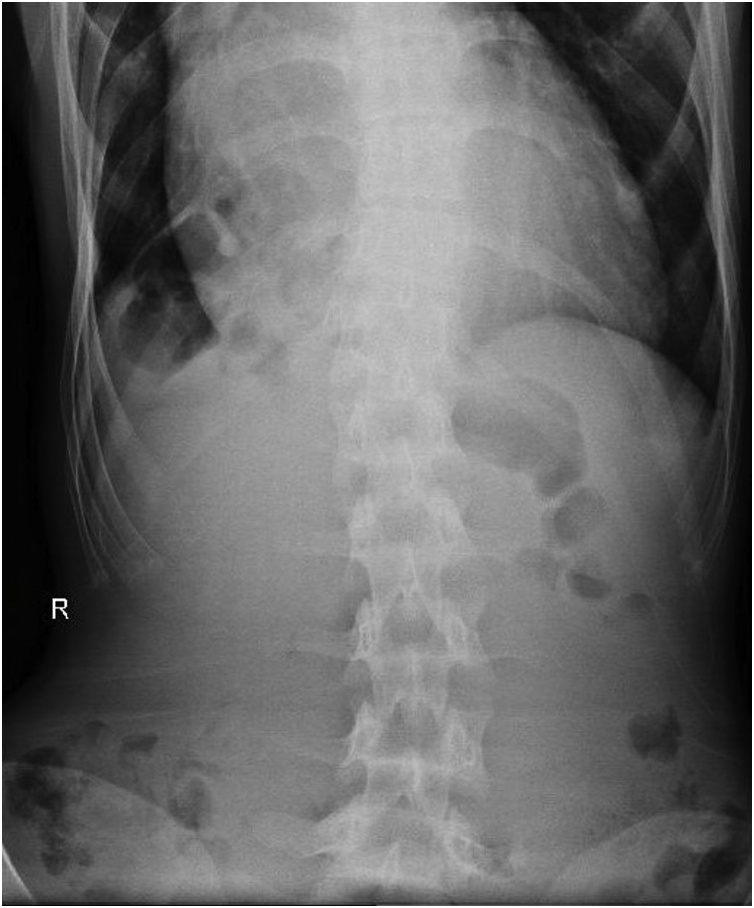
Plain abdominal radiograph suggesting the presence of subdiaphragmatic air.
